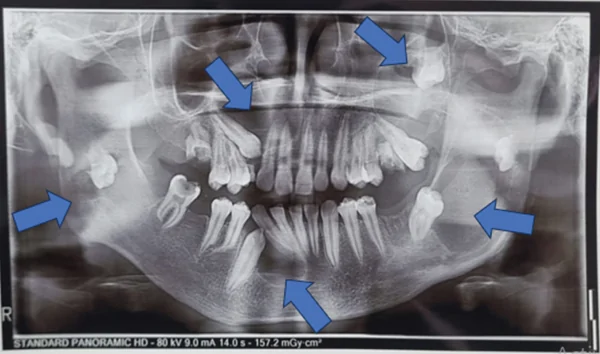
Panoramic radiograph showing multiple cystic lesions in Regions 13, 28, 38, 43, and 48.
